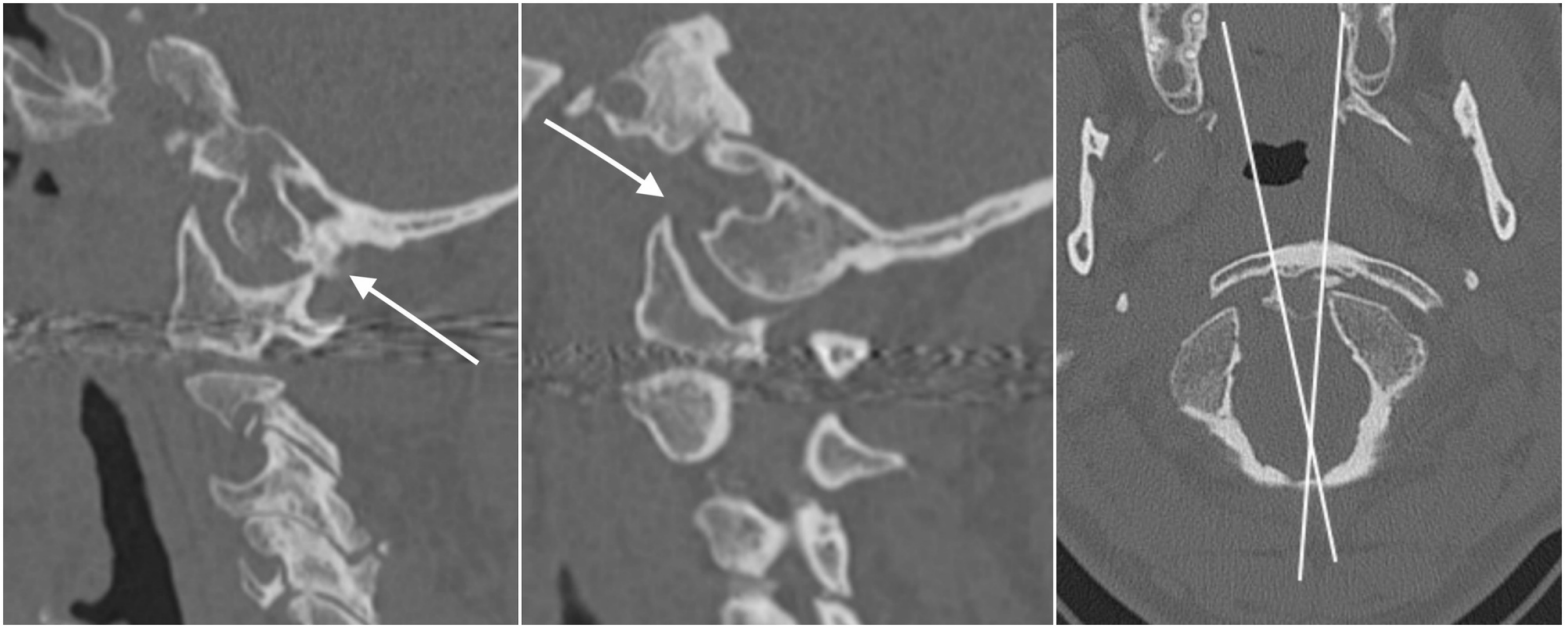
Computed tomography (CT) scan, atlanto-occipital rotatory dislocation Left, right parasagittal CT scan showing a widened condylar-C1 interval (2.5 mm) and a condylar fracture (arrow). Center, left parasagittal CT scan displaying widening of the condylar-C1 interval (4.3 mm). Right, axial CT scan showing rotatory displacement to the right of the occipital condyles over the atlas. The rotational angle is 13°.
